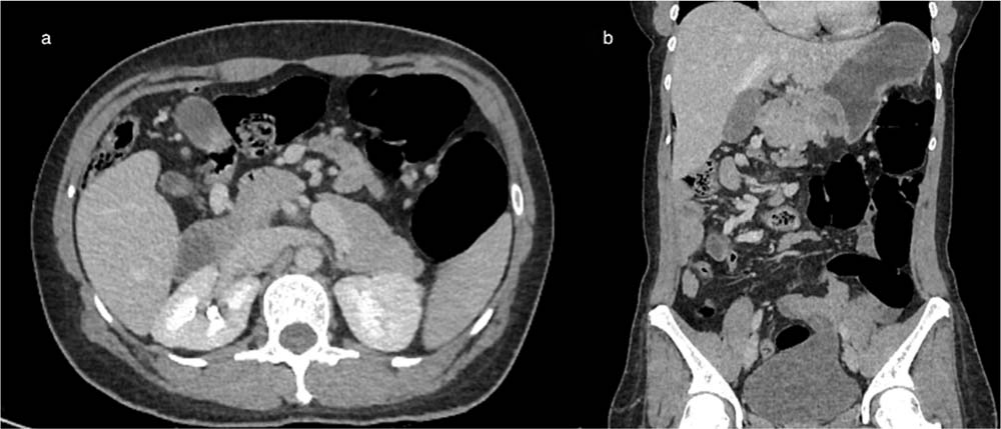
Axial (a) and coronal (b) contrast-enhanced CT images demonstrating intestinal malrotation, characterized by right-sided small bowel loops and left-sided colonic segments, with an abnormal relationship between the SMA and vein.
